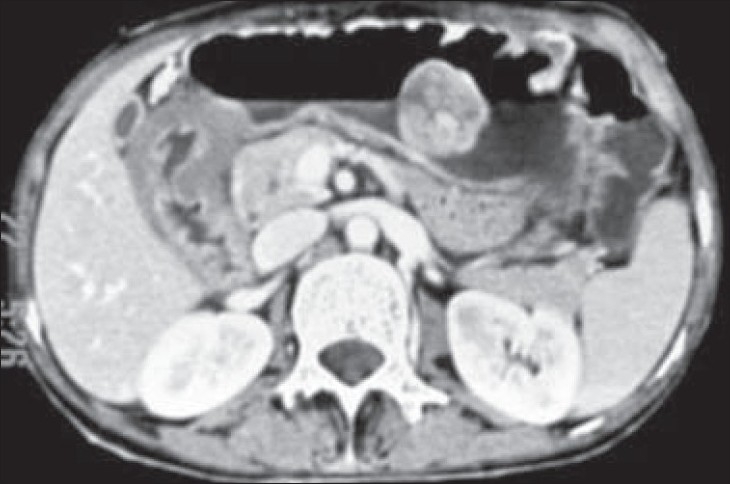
Axial contrast-enhanced CT image showing polypoidal neurofibroma along the greater curvature of the stomach and diffuse mural thickening in the region of pyloric antrum
